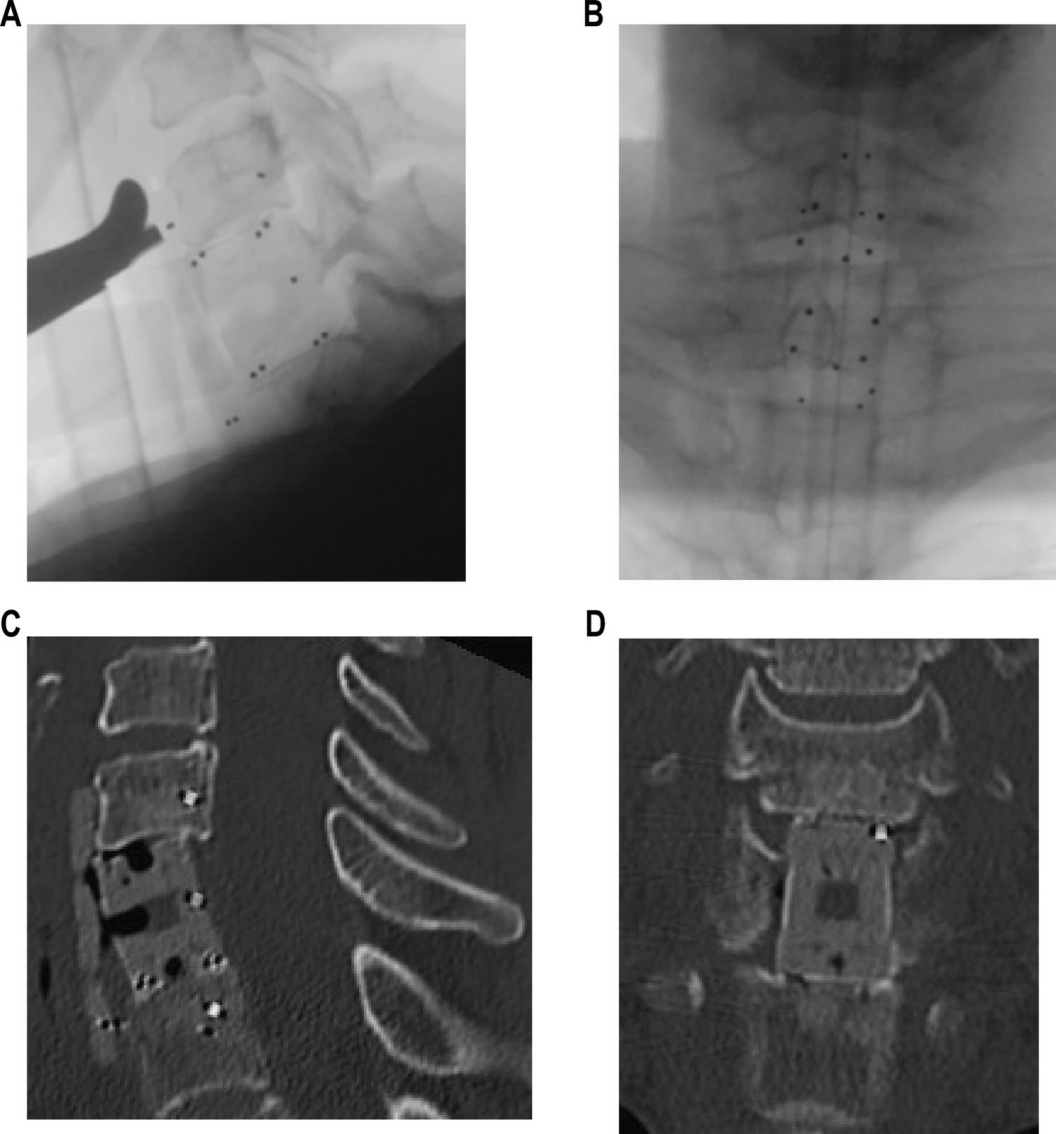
Case 1: Lateral (A) and a.p. (B) intraoperative X-ray controls after implantation of the CFRP cage. Postoperative CT controls in sagittal (C) and coronal view (D)
